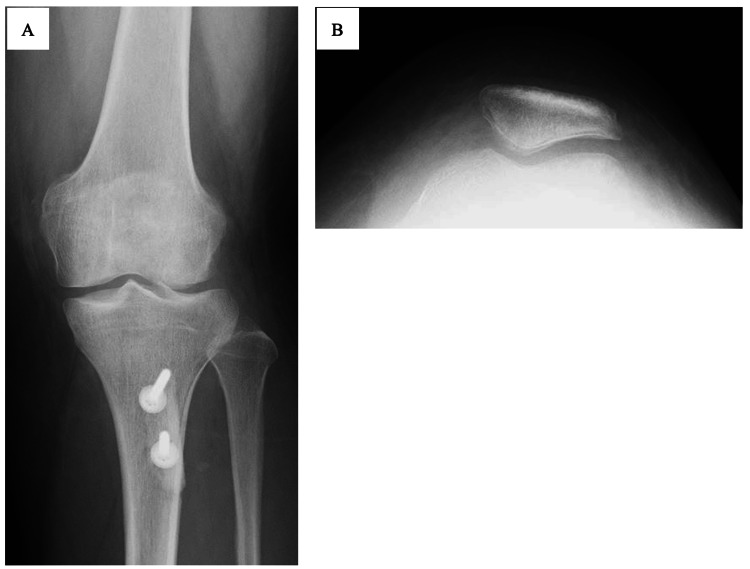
X-ray images of the 22-year-old female patient before hardware removal and medial patellofemoral ligament reconstruction Radiographic images of the left knee joint after tibial tuberosity osteotomy and medial patellofemoral ligament augmentation (A). The patella is in its normal position at 60° knee flexion (B).
